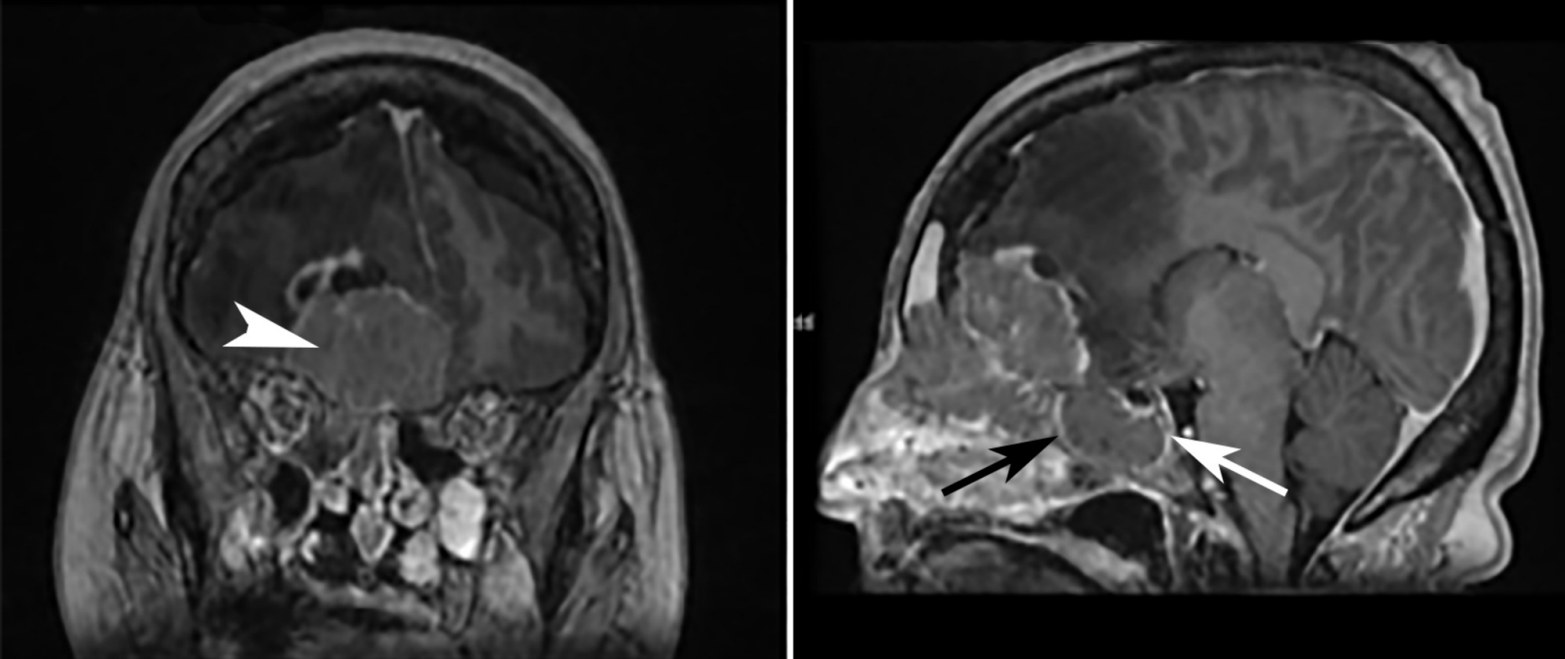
MRI head, T1 coronal and sagittal Coronal (*left*) and sagittal (*right*) T1-weighted post-contrast reformats demonstrate intracranial extent of the mass (*arrowhead*). In addition to the previously noted areas of involvement, rim-enhancing soft tissue signal is also seen involving the sphenoid sinus (*arrows*). MRI: magnetic resonance imaging
